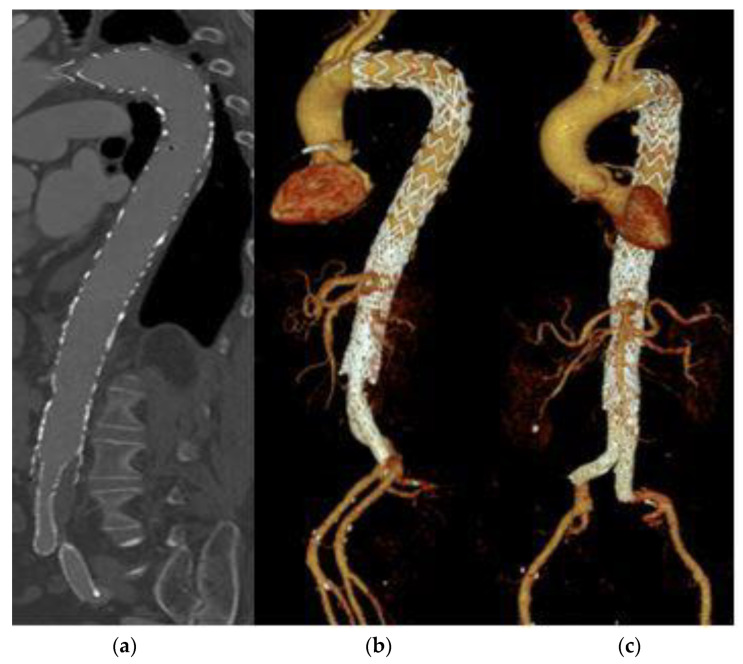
Aortic dissection after stent implantation and Y-prosthesis. (a) CT view from lateral, (b,c) show a 3D visualization of the entire aorta from lateral and ventral view respectively.
